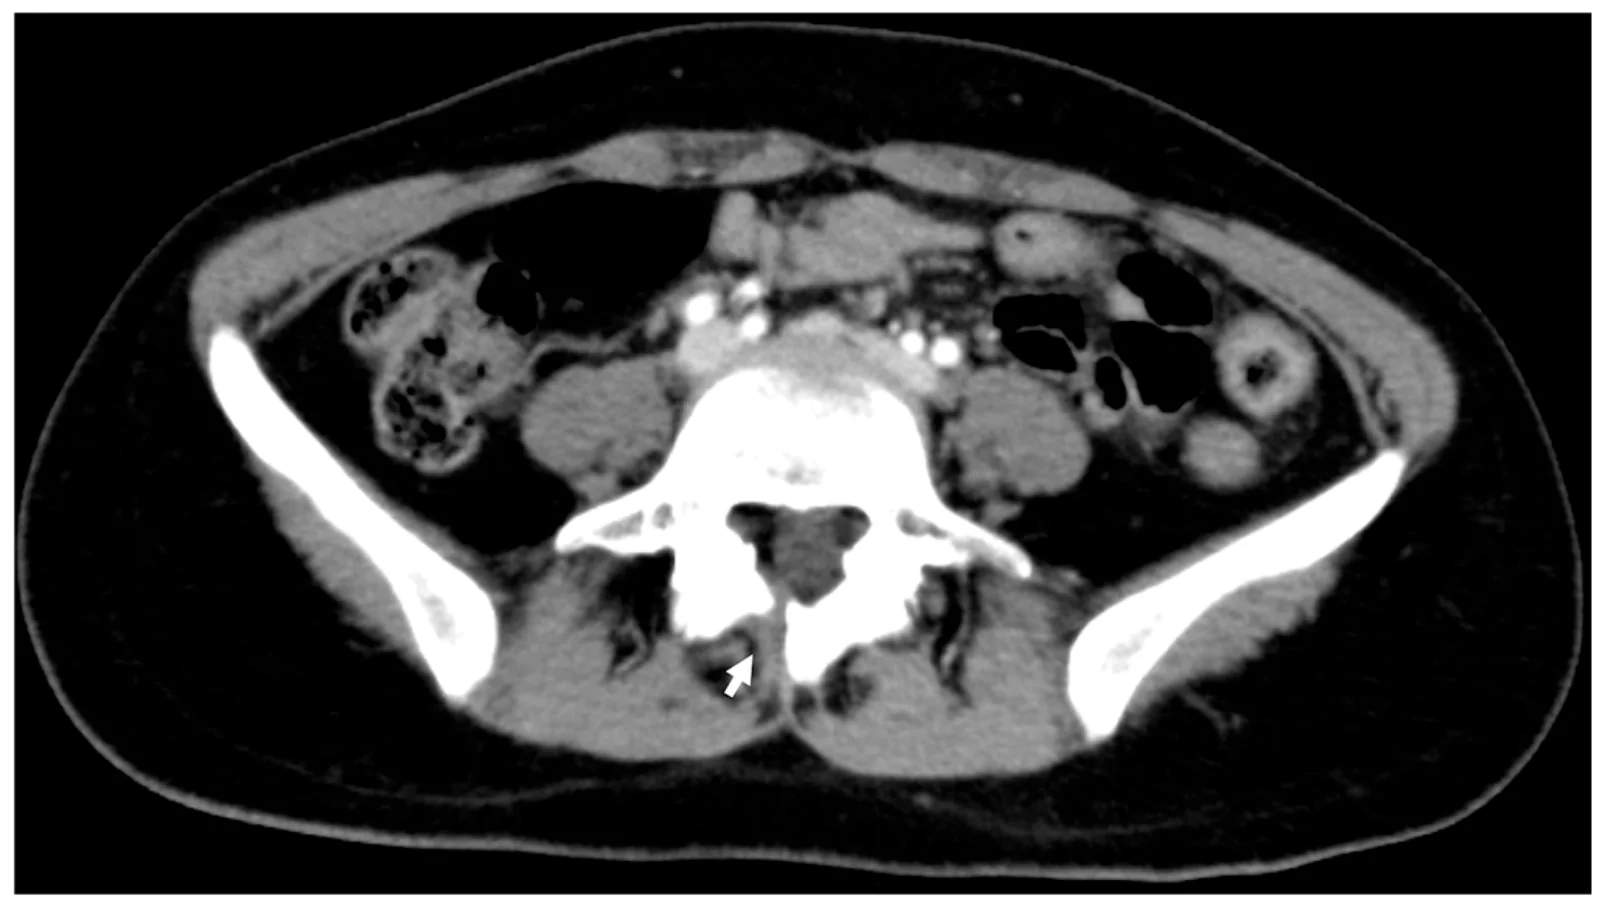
Lumbar computed tomography.
